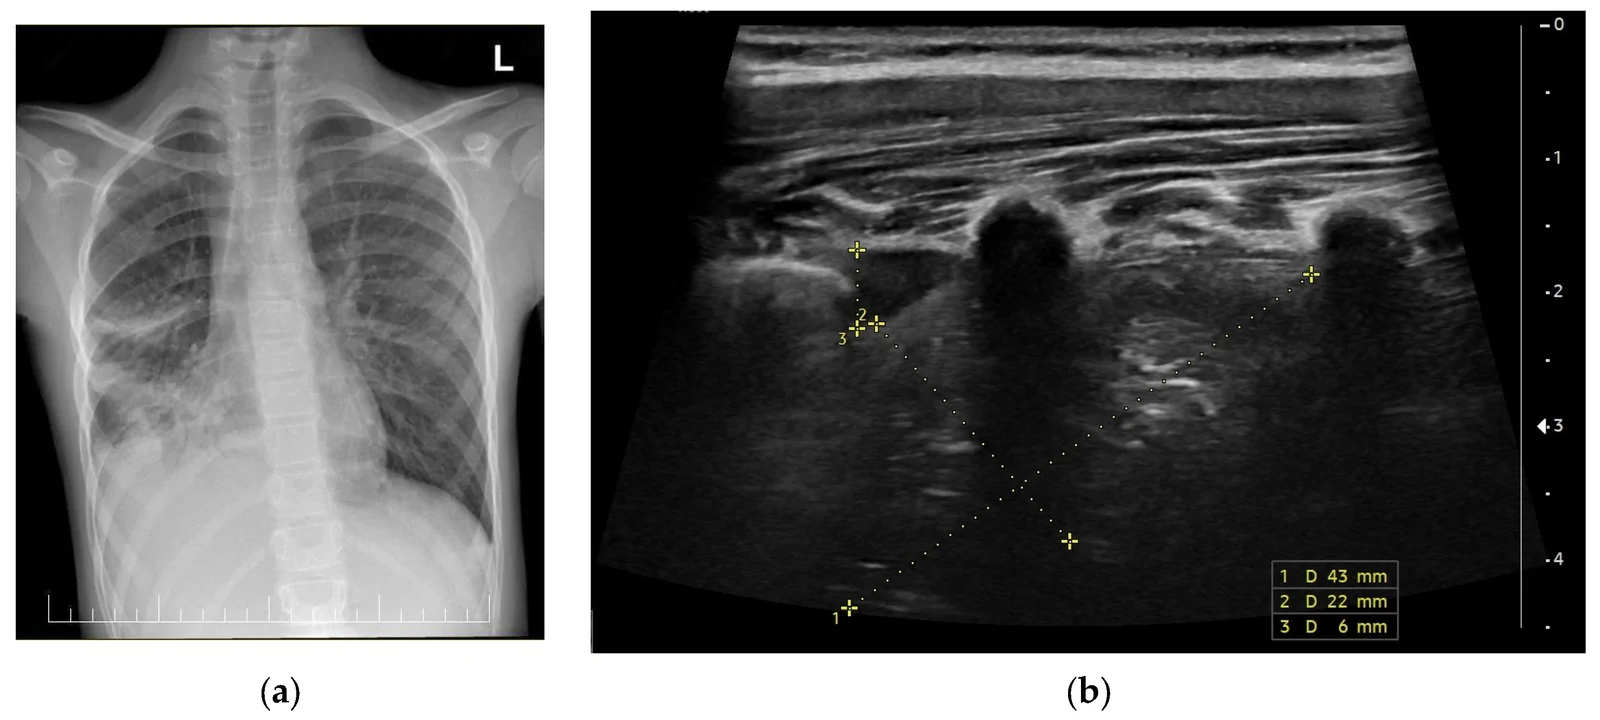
(a) Posteroanterior chest radiograph of a 10-year-old female demonstrating bilateral pneumonia with right-sided pleural effusion. (b) Lung ultrasound of the same patient reveals pulmonary consolidation and adjacent pleural effusion.
